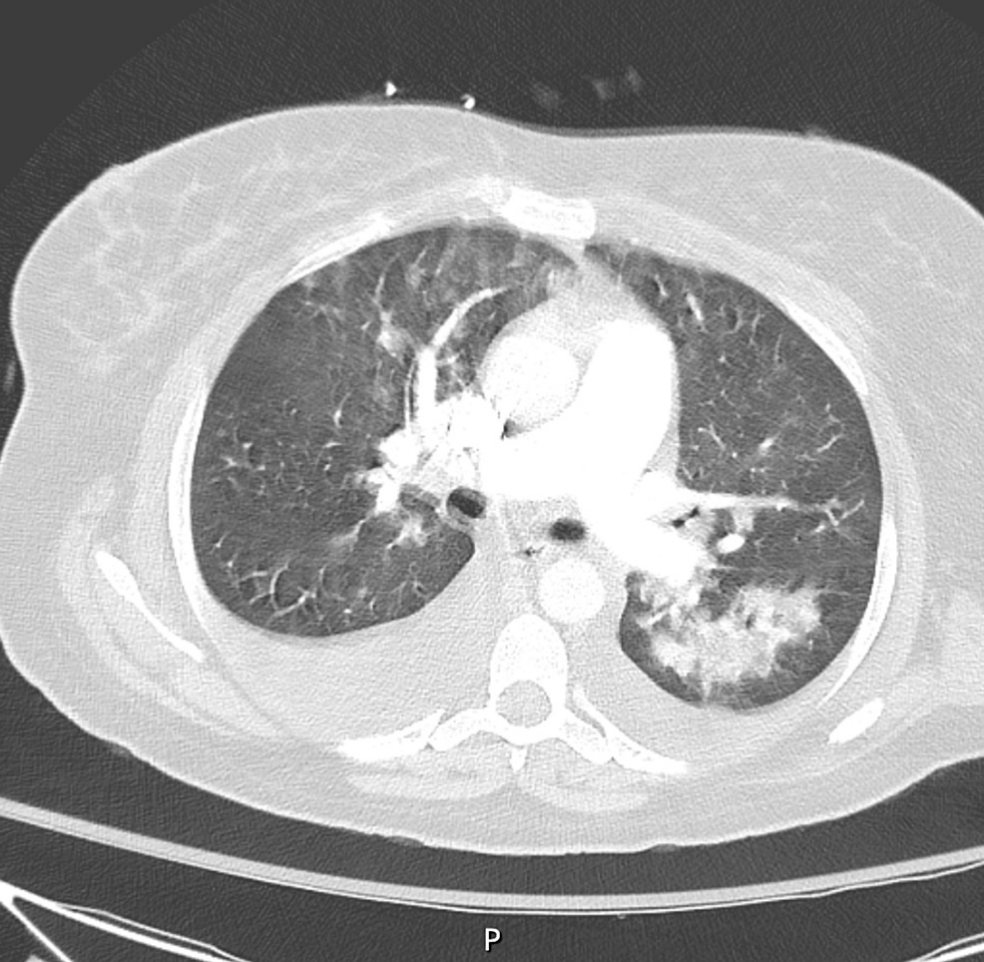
CT angiogram chest Bifurcation of pulmonary artery
